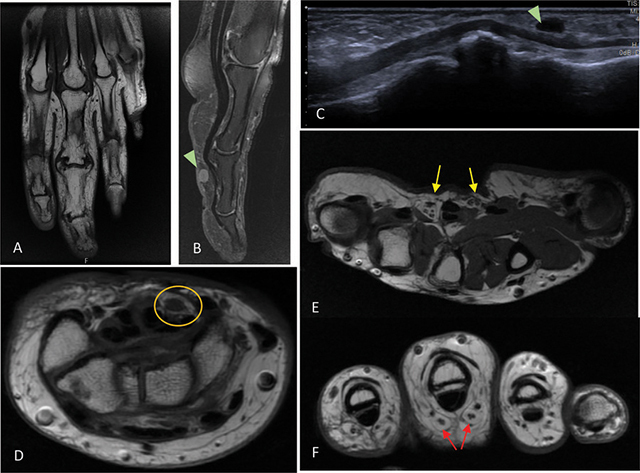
Hand MRI. (A–B) Coronal T1-weighted and sagittal PDFS images showing lipomatous hypertrophy of the subcutaneous tissue of the third finger, predominant on the volar side. Small synovial cysts are present (Fig. 2B) and coincide with anechoic structures on ultrasound (Fig. 2C). (D) Axial T1-weighted image with no significant abnormalities of the median nerve (orange circle) and no space-occupying lesions. Axial T1-weighted images through the metacarpal heads (E) and proximal phalanx (F) show subtle fatty infiltration of the common volar digital nerves (yellow arrow) and proper volar digital nerves (red arrows). PD—proton density. FS—fat-saturated.
